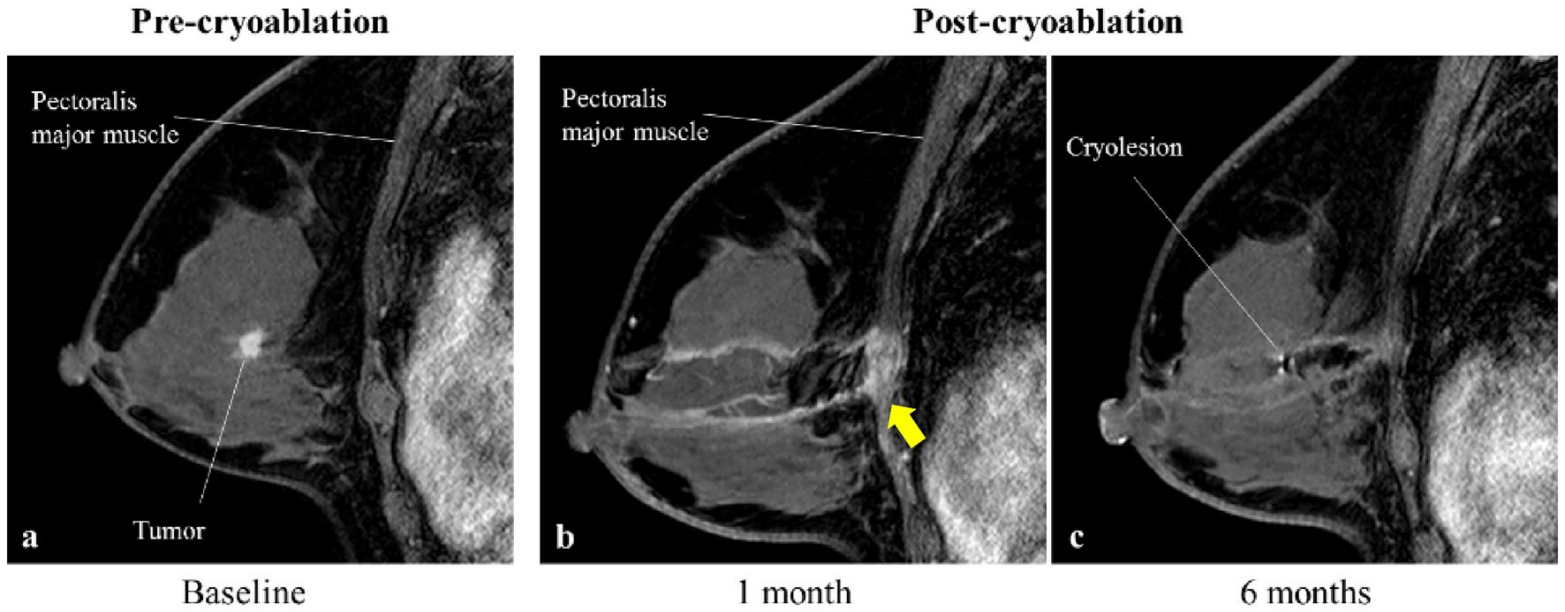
Pre- and post-cryoablation MRI images—pectoralis major muscle burn. MRI images pre- (a) 1 month (b) and 6 months (c) post-cryoablation in a patient 55 years old with 8.9 mm tumor in the left breast. Arrow points to the area of the pectoralis major muscle burns
